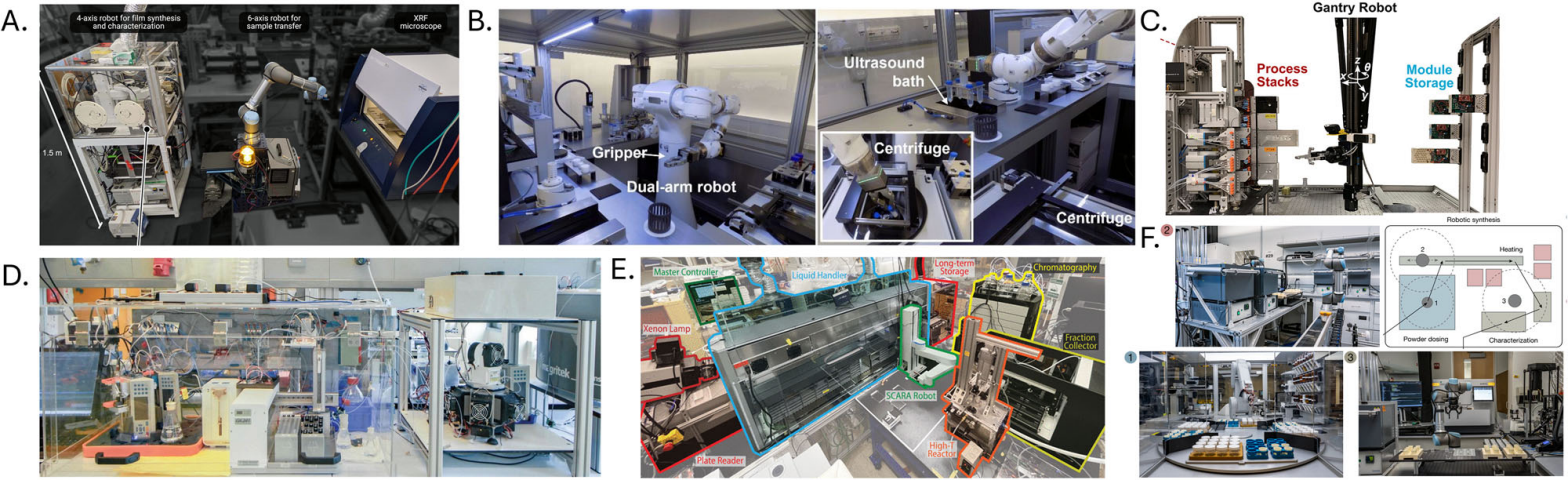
Robotic systems as integrators in lab automation. A Four- and six-axis robotic platforms for autonomous materials synthesis and sample transfer. B Dual-arm robotic system integrating ultrasound and centrifugation for automated sample preparation. C Gantry-based robot enabling modular, multistep flow synthesis. D Automated platform for closed-loop optimisation of photocatalytic reactions. E Integrated system combining robotic synthesis with computational modelling for molecular discovery. F Robotic laboratory platform supporting heating, dosing and in situ characterisation. Panels reproduced with permission from MacLeod et al. (2022), Dembski et al. (2023) and Szymanski et al. (2023) under CC BY licences; from Nambiar et al. (2022) with permission from the American Chemical Society; and from Slattery et al. (2024) and Koscher et al. (2023) with permission from the American Association for the Advancement of Science (AAAS).
